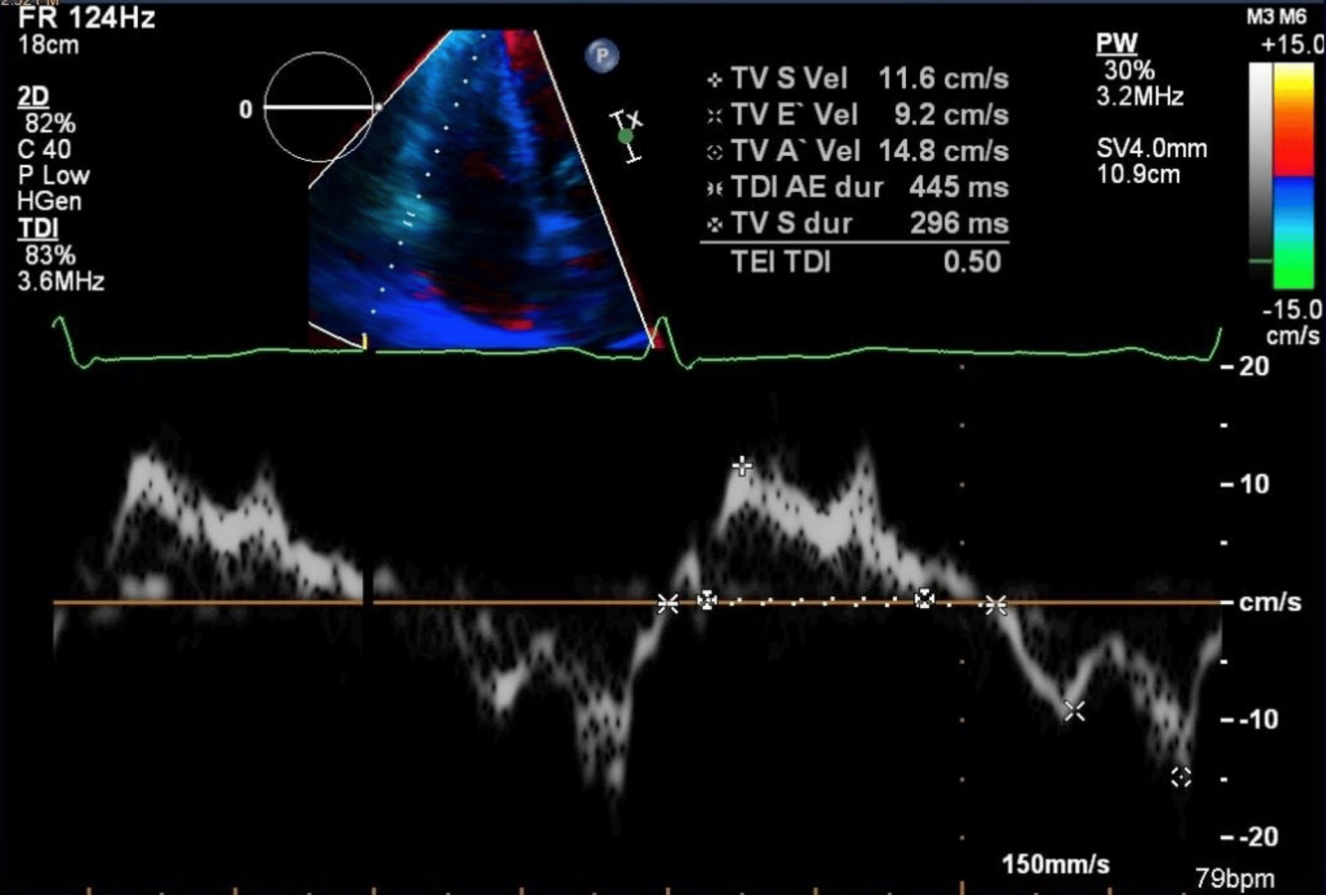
Pulsed-wave TDI of the lateral tricuspid annulus in an apical four-chamber view TDI: tissue Doppler imaging; S': systolic; E': early diastolic; A': late diastolic; TV: tricuspid valve The spectral Doppler trace displays the S’, E’, and A’ annular velocities, reflecting right ventricular longitudinal myocardial function. The measured velocities include TV S’ 11.6 cm/s, TV E’ 9.2 cm/s, and TV A’ 14.8 cm/s, respectively. Time intervals for isovolumic contraction, ejection, and relaxation are shown, allowing calculation of the right ventricular myocardial performance index (Tei index = 0.50). These measurements correspond to borderline-normal systolic function of the right ventricle
